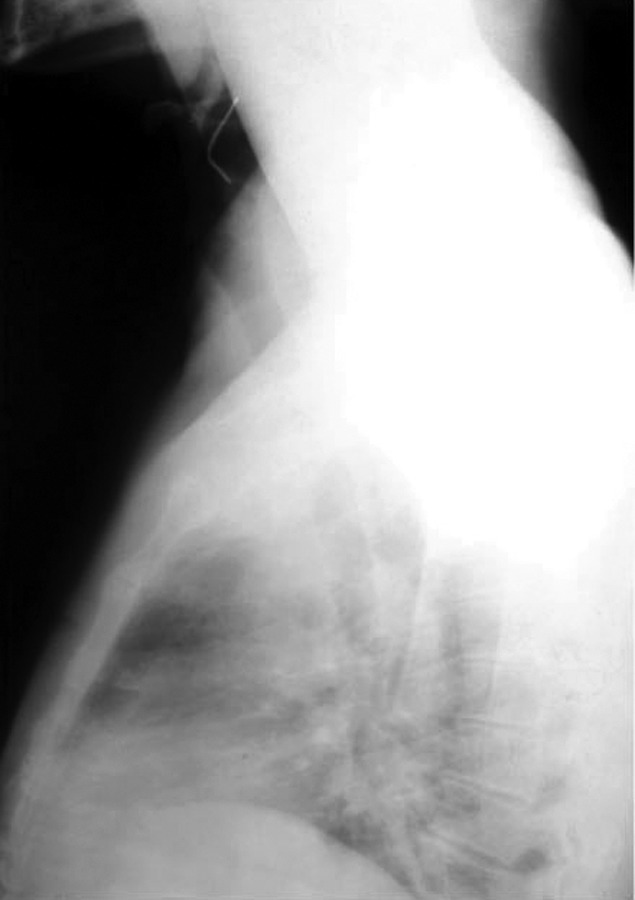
Roentgenogram of cervical region showing the ‘bend’
